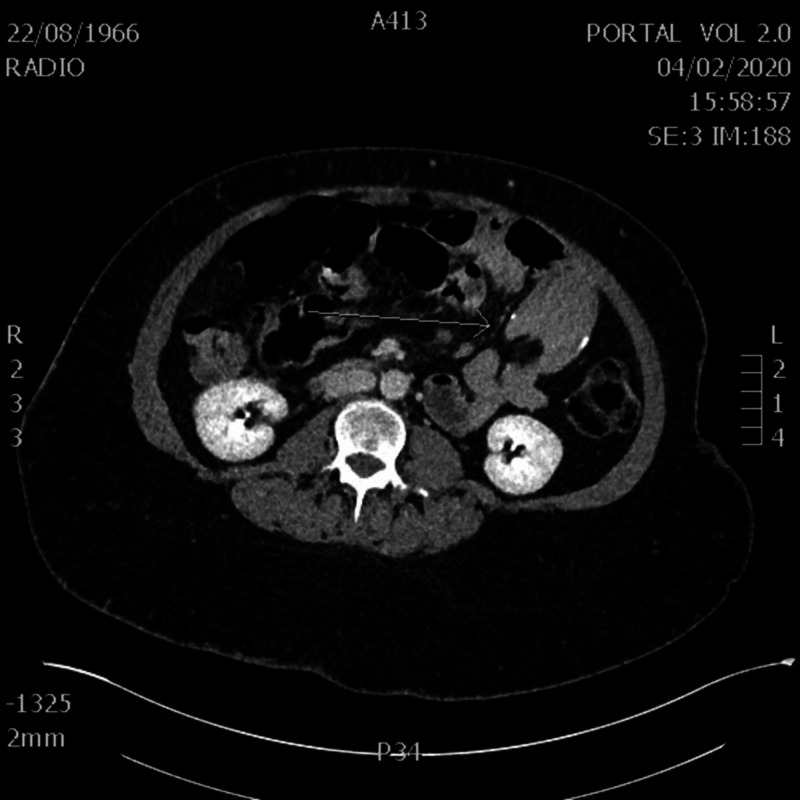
Axial view of the CT scan showing intussusception at level of jejuno-jejunal anastomosis
